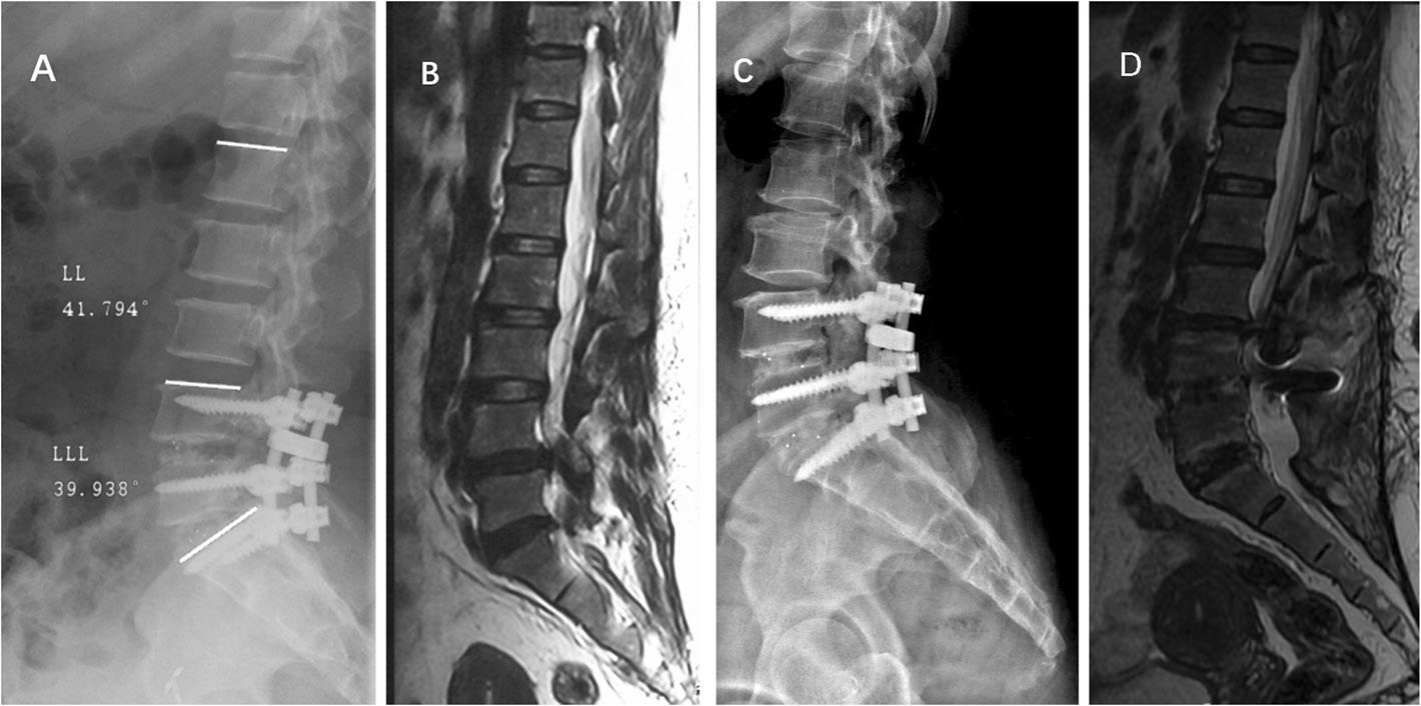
Imaging studies of ASD associated with high postoperative LDI. a A 47-year-old female patient underwent L4-S1 PLIF with the postoperative LL of 41.79° and LLL of 39.94°. The ultimate LDI equals to 95.57%. b Preoperative sagittal T2-weighted MRI scans at the L3–4 showing no or mild spinal stenosis (Imagama’s classification II) and disc degeneration of Pfirrmann classification III. c Postoperative neutral lateral X-ray obtained 69 months after surgery revealing retrolisthesis of L3 vertebra. d The final follow-up MRI scans demonstrating severe spinal stenosis (Imagama’s classification IV) and disc degeneration of Pfirrmann classification IV
